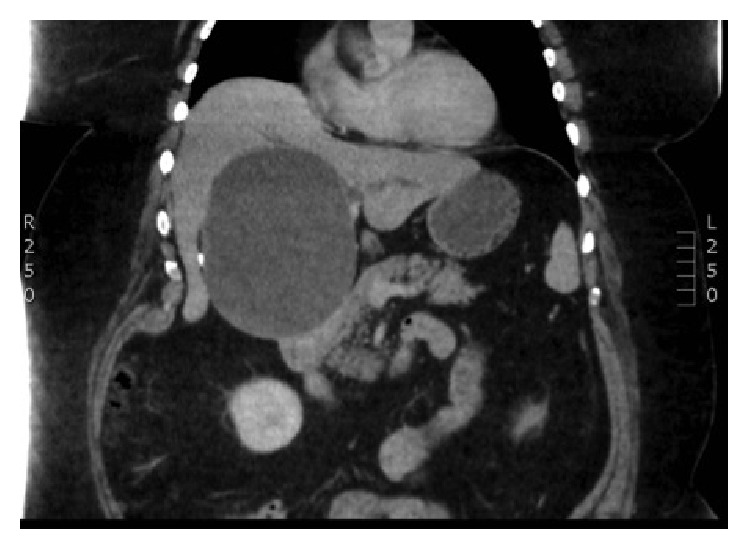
CT scan showing unilocular biliary cystadenoma without any septations but with calcification in the wall.
